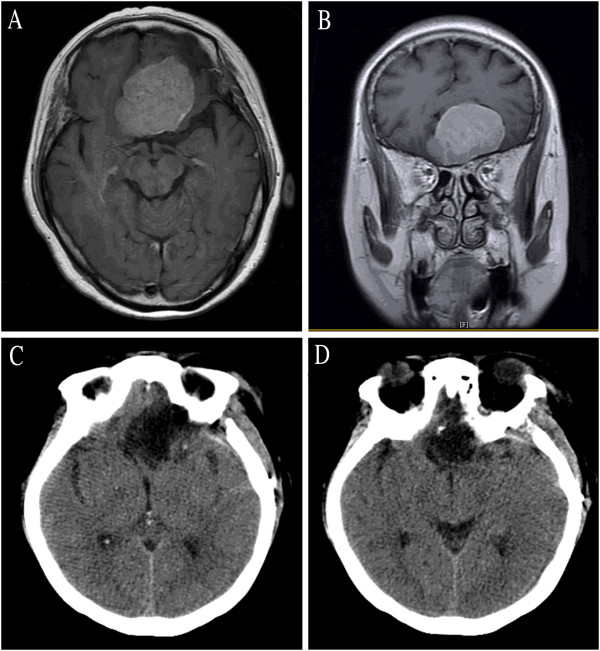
Case number 20: Axial (A), and coronal (B) view of contrasted magnetic resonance imaging showing a tuberculum sellae meningioma. (C-D) Postoperative CT imaging of the same patient.
